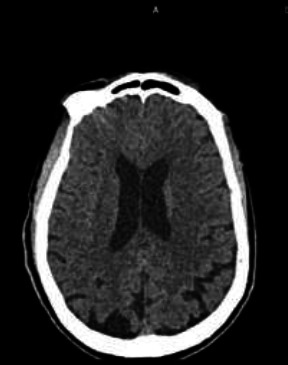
A Plain CT brain done on recurrence showing no haemorrhage or acutely demarcated infarct
